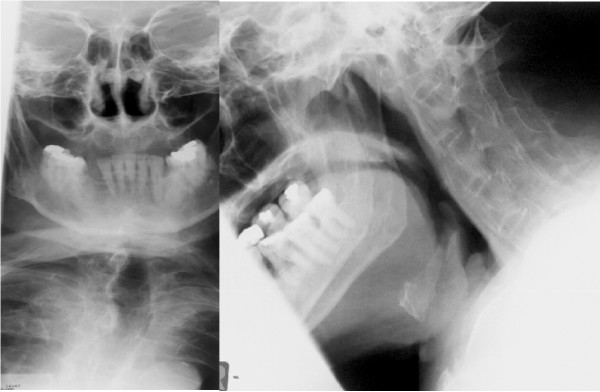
X-Ray's in standard plain show a reduced view of the lower cervical spine and of the cervico-thoracic junction. Furthermore, an accurate evaluation is difficult due to the ossification and osteoporosis.
